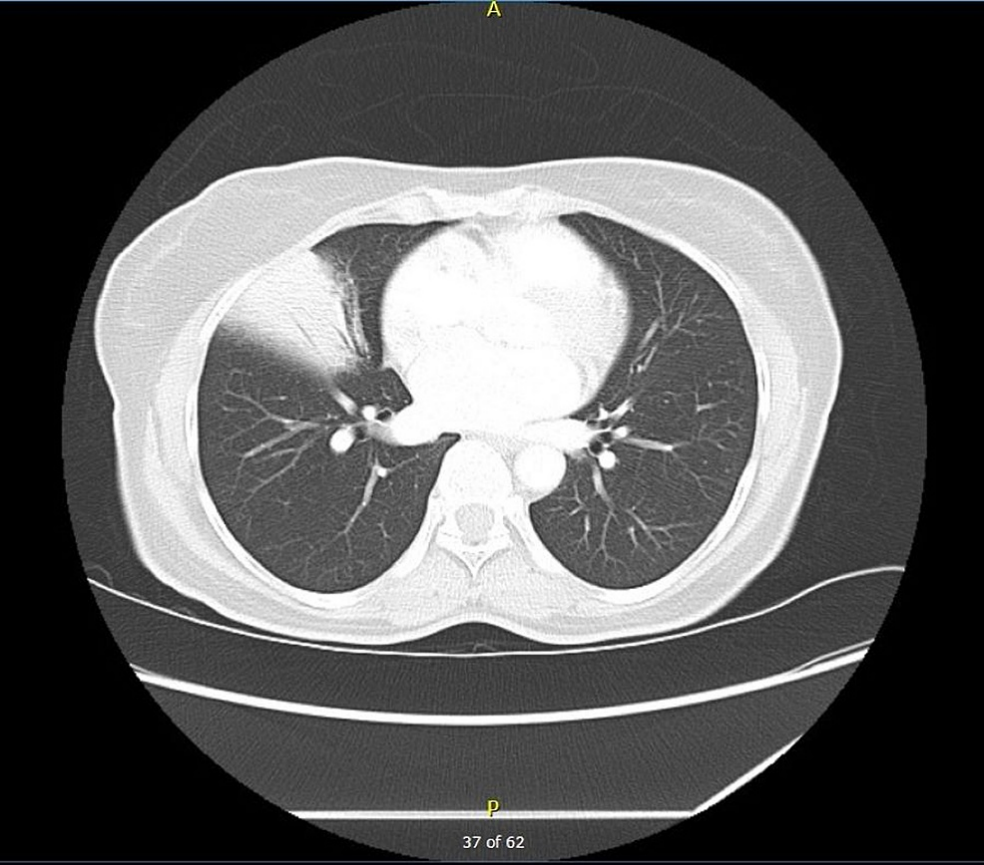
Initial CT chest was remarkable for right middle lobe opacity with air bronchogram.
